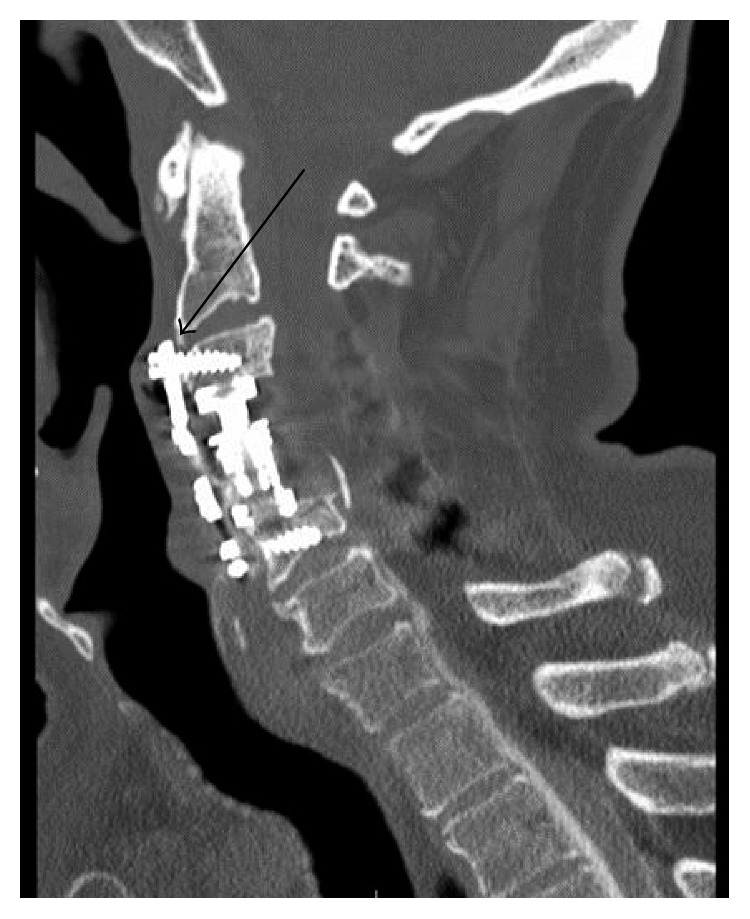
CT neck showing cervical hardware with thinning of the oropharyngeal mucosa. CT myelogram was negative for leak in this region.
